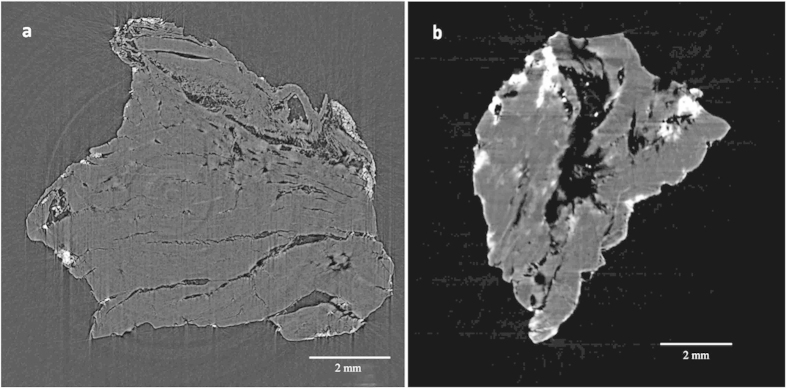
The CT slices of the cross section of stick 1 (a) and stick 2 (b).
